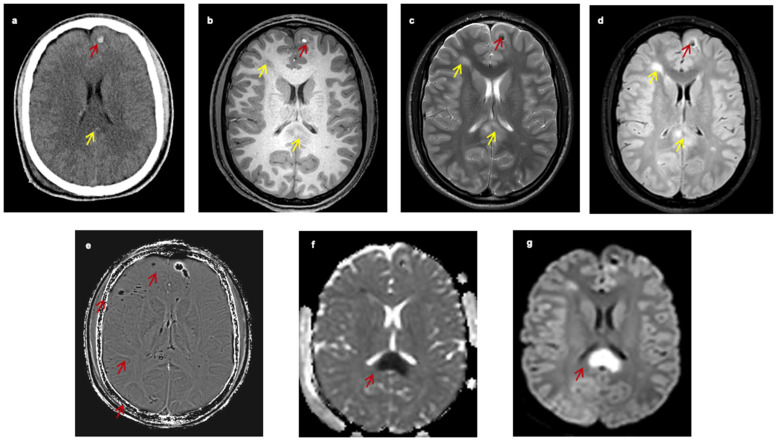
17-Year-old male with traumatic axonal injury (unpublished data) (a) CT scan on admission shows hyperdense foci (microhaemorrhage) in the gray–white matter junction of the left frontal lobe (red arrow) and hypodensity of the splenium of the corpus callosum (yellow arrow). (b) MRI, three days later, axial T1WI shows a hyperintense lesion at the gray–white matter junction of the left frontal lobe compatible with subacute hemorrhage (red arrow) and hypointense lesions in the deep white matter of the right frontal lobe and the slpenium (yellow arrow). (c) MRI axial T2WI, and (d) FLAIR images show the left frontal lobe lesion with low signal (red arrow) and the lesions of the deep white matter of the right frontal lobe and the splenium with high signal intensity (yellow arrow). (e) MRI axial SWI sequence shows multiple low signal intensity lesions compatible with haemorrhage mainly located in the gray–white matter junction of fronto-occipital lobes and the right side of the splenium of corpus callosum (red arrow). (f) DWI image (g) and ADC parametric map reveal restricted diffusion within the splenium of the corpus callosum (red arrows), compatible with cytotoxic oedema. Key: CT, computed tomography; MRI, magnetic resonance imaging; WI, weighted imaging; SWI, susceptibility-weighted imaging; DWI, diffusion-weighted imaging.
